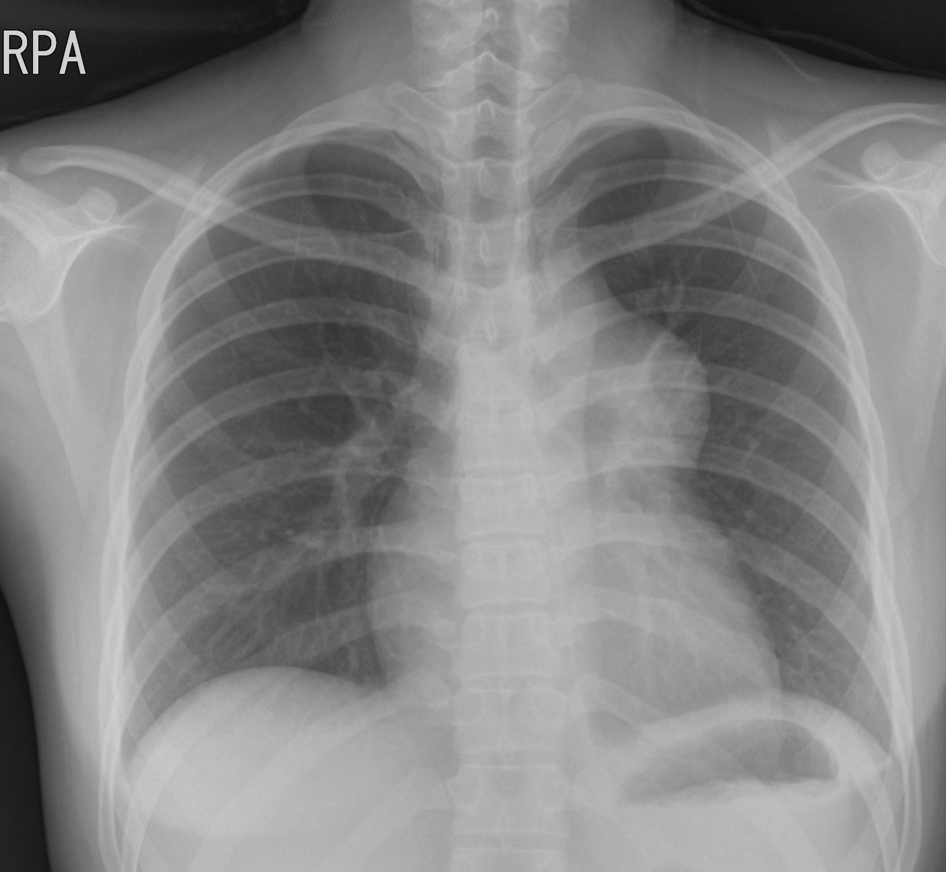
Chest X-ray showing protruding well-defined mass shadow in the left upper hilar space.
